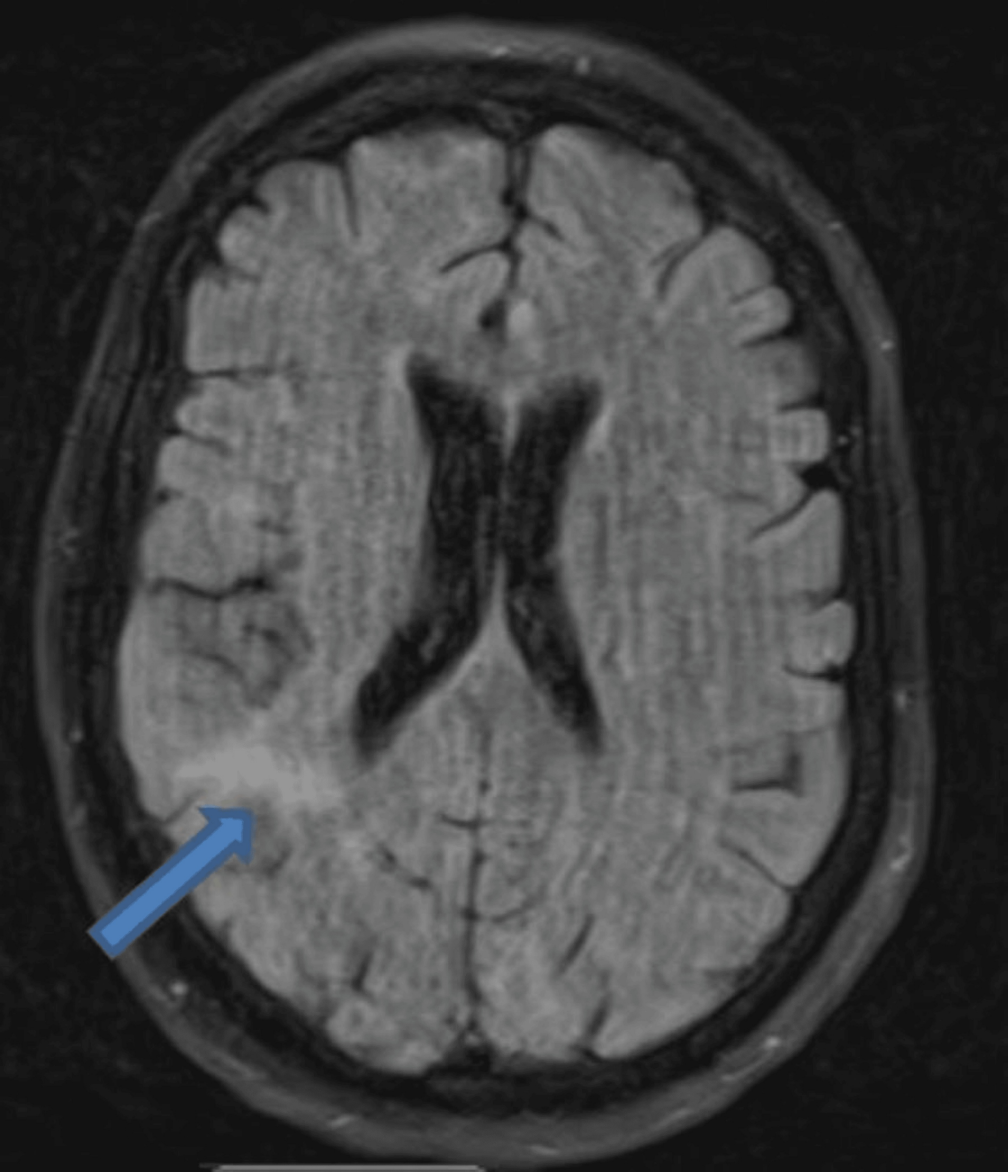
Head MRI T1 image with contrast (axial view): increasing diffusion restriction in white matter around the right parietal gyrus and parietal lobe (arrow)
